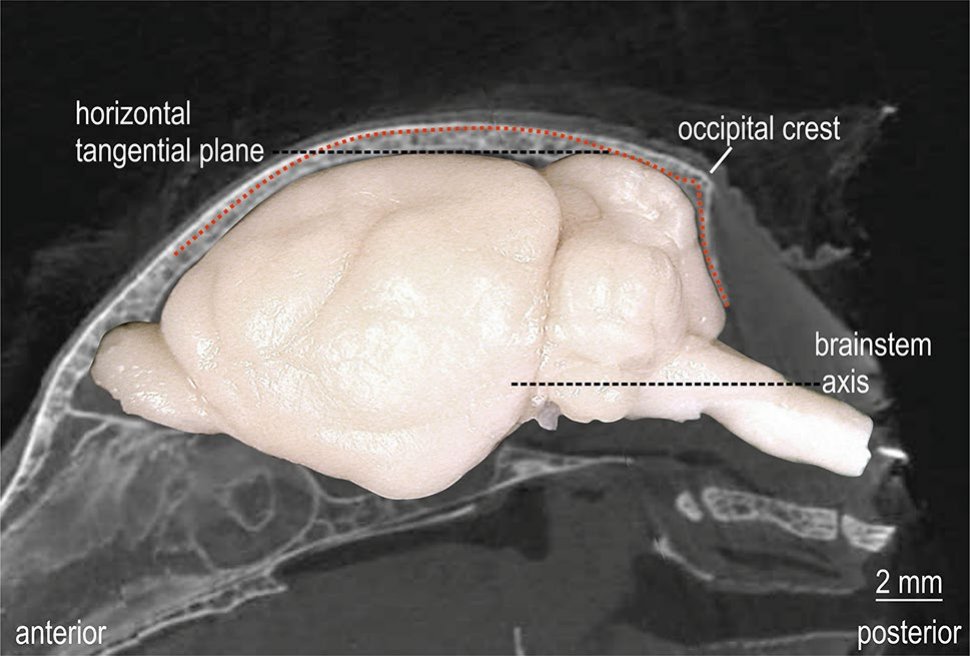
Atlas coordinate system and stereotaxic reference system. Montage of CT image of mid-parasagittal skull with the abrupt decline of occipital crest and the side-view image of the brain in standard orientation of the atlas. The red dotted line corresponds to the outer skull profile of the parasagittal CT image 1000 µm lateral to midline. The horizontal tangential plane through the most dorsal points of cerebrum and cerebellum and the brain stem axis is indicated by black dashed lines
